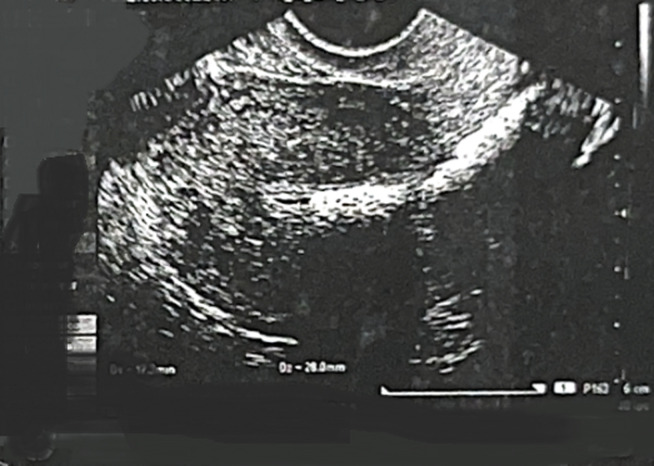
trans-vaginal ultrasound scan showing dense linear hyperechoic substances in the endometrium with acoustic shadowing
